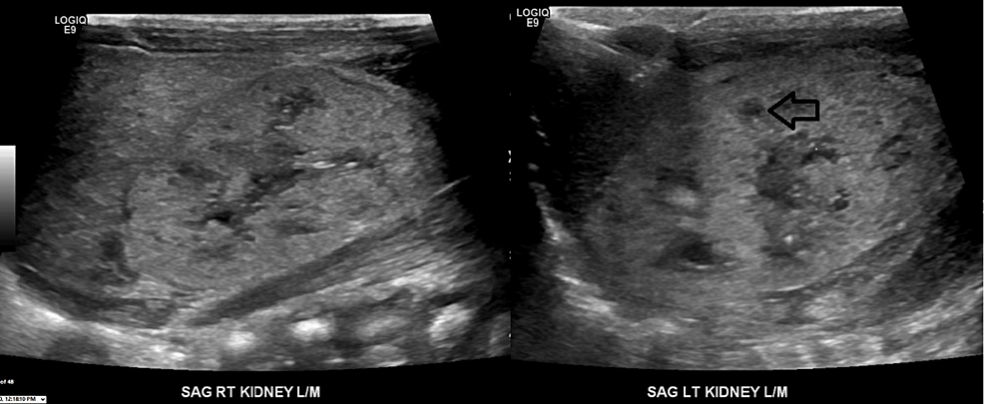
Echogenic kidney and small cyst (black arrow) in renal ultrasound of the left (LT) kidney
